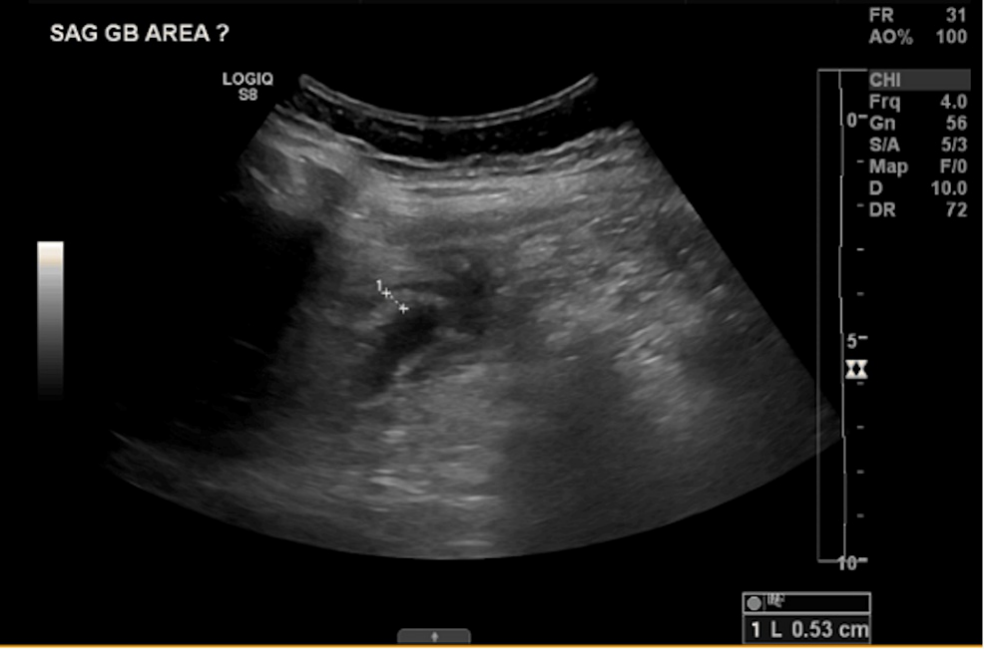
Abdominal ultrasound showing thickening of gallbladder wall of 53 mm
